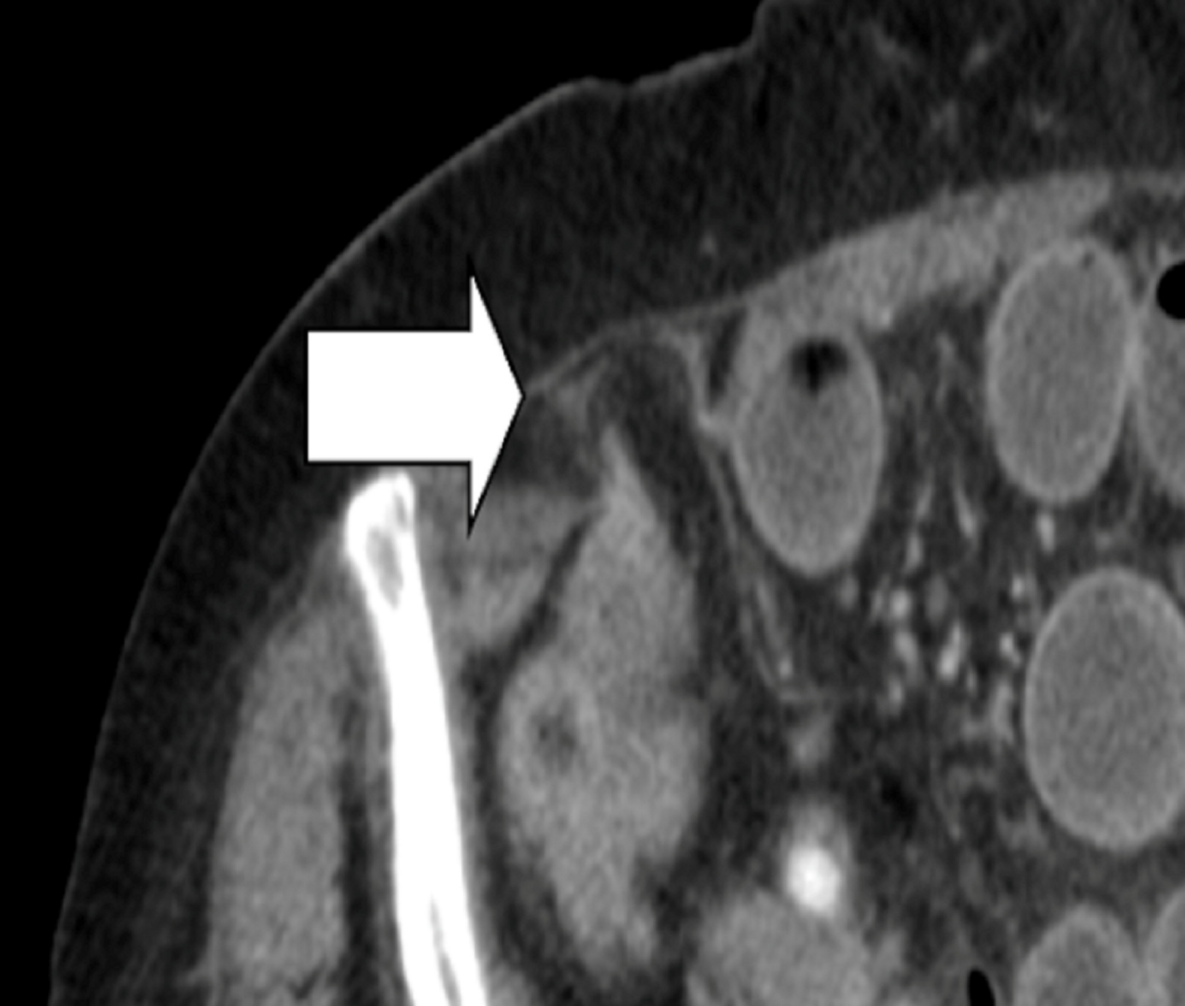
Zoom-in of the computed tomography (CT) scan showing a Spigelian hernia defect
